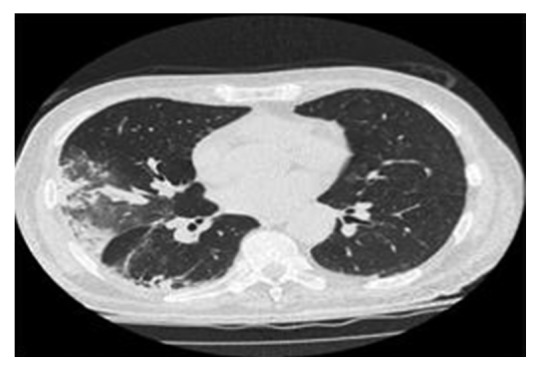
Chest CT scan showing bilateral ground glass opacities and nodular densities.
